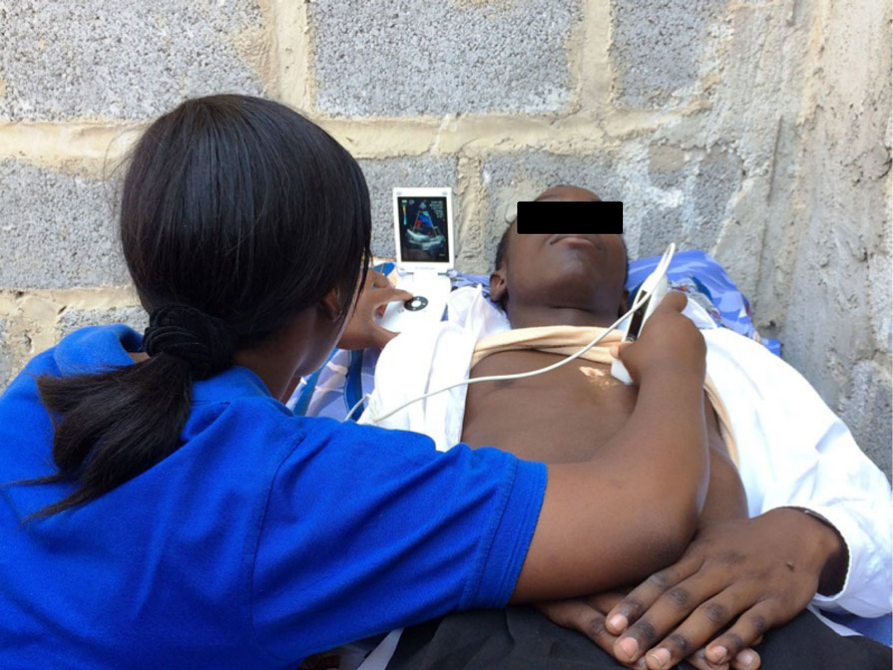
A radiographer from Lusaka University Teaching Hospital uses ultraportable echocardiography to screen an adolescent for rheumatic heart disease as part of the study (photograph used with permission of participant)
